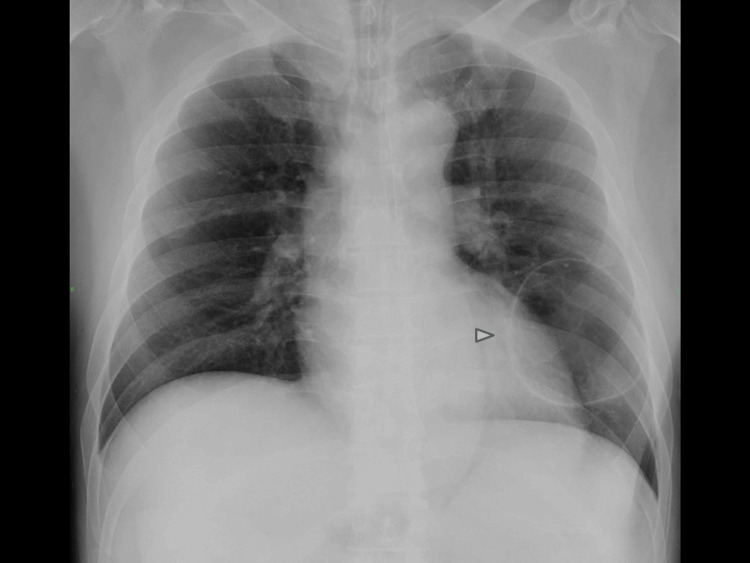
Chest radiograph on admission (Day 0). Post-intubation AP chest X-ray shows a large, well-circumscribed, thin-walled lucent cystic lesion in the left lower lung field (arrowhead). AP: anteroposterior projection.
